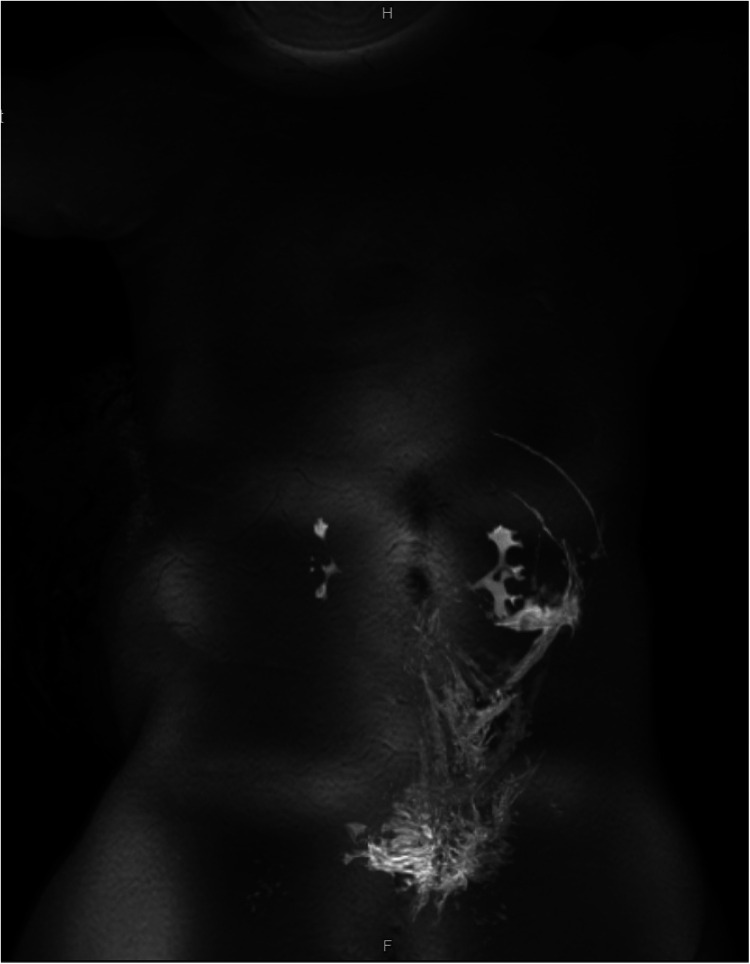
MRI lymphangiogram. Majority of contrast spreads along the left retroperitoneal and perirenal space.
